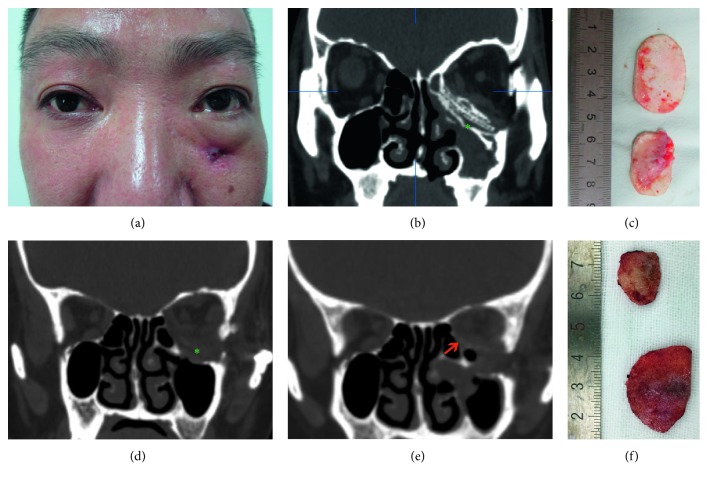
Two cases of implant infections. (a) Patient with infraorbital intermittent swelling and fistula 9 years after implantation of hydroxyapatite. (b and d) CT showing intraorbital abscesses in these two cases (asterisks). (c and f) The explanted implants. (e) Orbital walls were secondarily reconstructed using Rapidsorb in a one-stage procedure with removal of the previous implants (red arrow).
